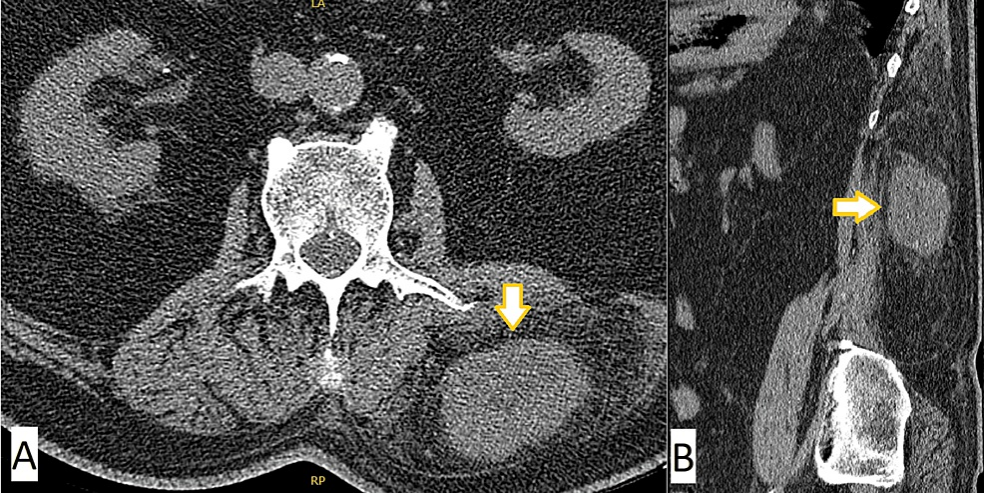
A: Axial CT image of left paraspinal mass superficial to musculature B: Sagittal CT image of mass
